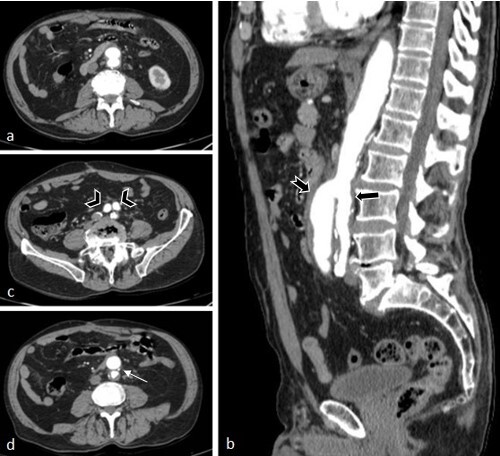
(a) Axial computed tomography (TC) images with an abdominal window; (b) Sagittal CT image with an abdominal window showing inferior duplication of the abdominal aorta with an anterior unit (tailed arrow) and a posterior unit (simple arrow), from the level of L3; (c) Axial CT image with an abdominal window showing inferior duplication of the abdominal aorta, with an anterior unit with a caliber of 2.6 cm, bifurcating into the external iliac arteries (arrowhead); and a posterior unit with a caliber of 2.2 cm, bifurcating into internal iliac arteries (thrombosed in this study); (d) Axial CT image with an abdominal window showing duplication of the inferior portion of the abdominal aorta, with the posterior unit giving rise to the inferior mesenteric artery (thin arrow).
